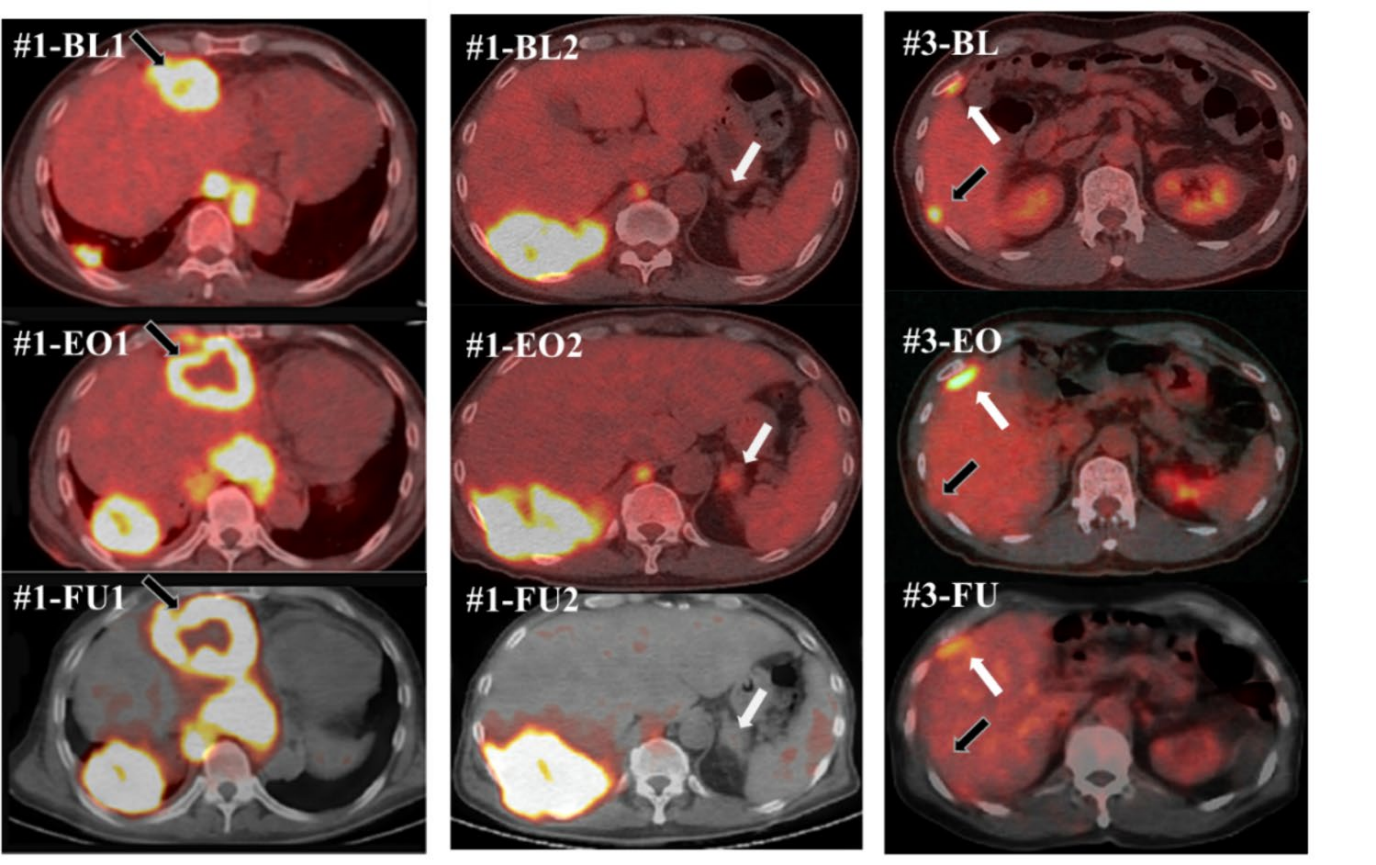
Representative PET/CT scan imaging of the tumors that respond well after being treated with HVJ-E in patients with chemotherapy-resistant MPM
